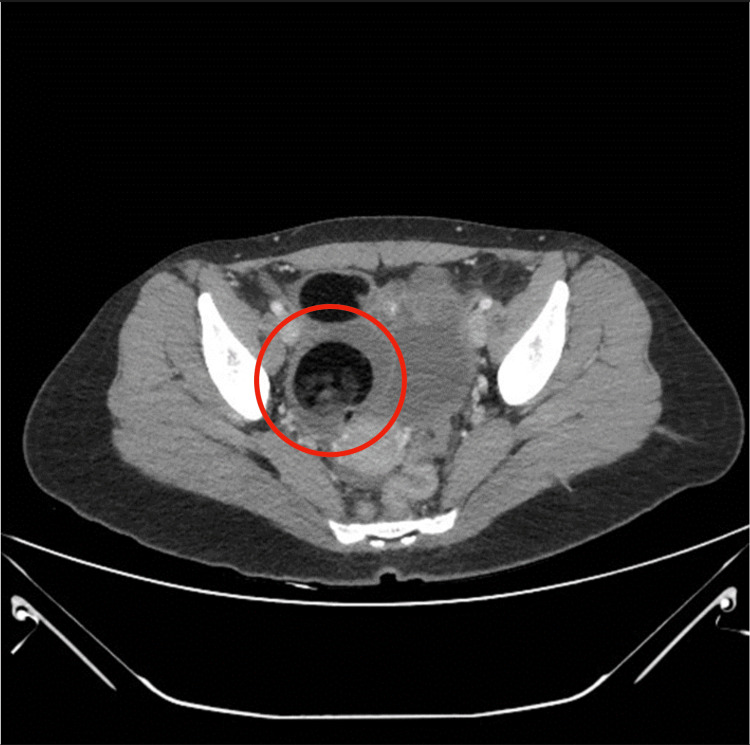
Computed tomography of the abdomen and pelvis showing a 10-cm mass in the right lower quadrant (red circle)
